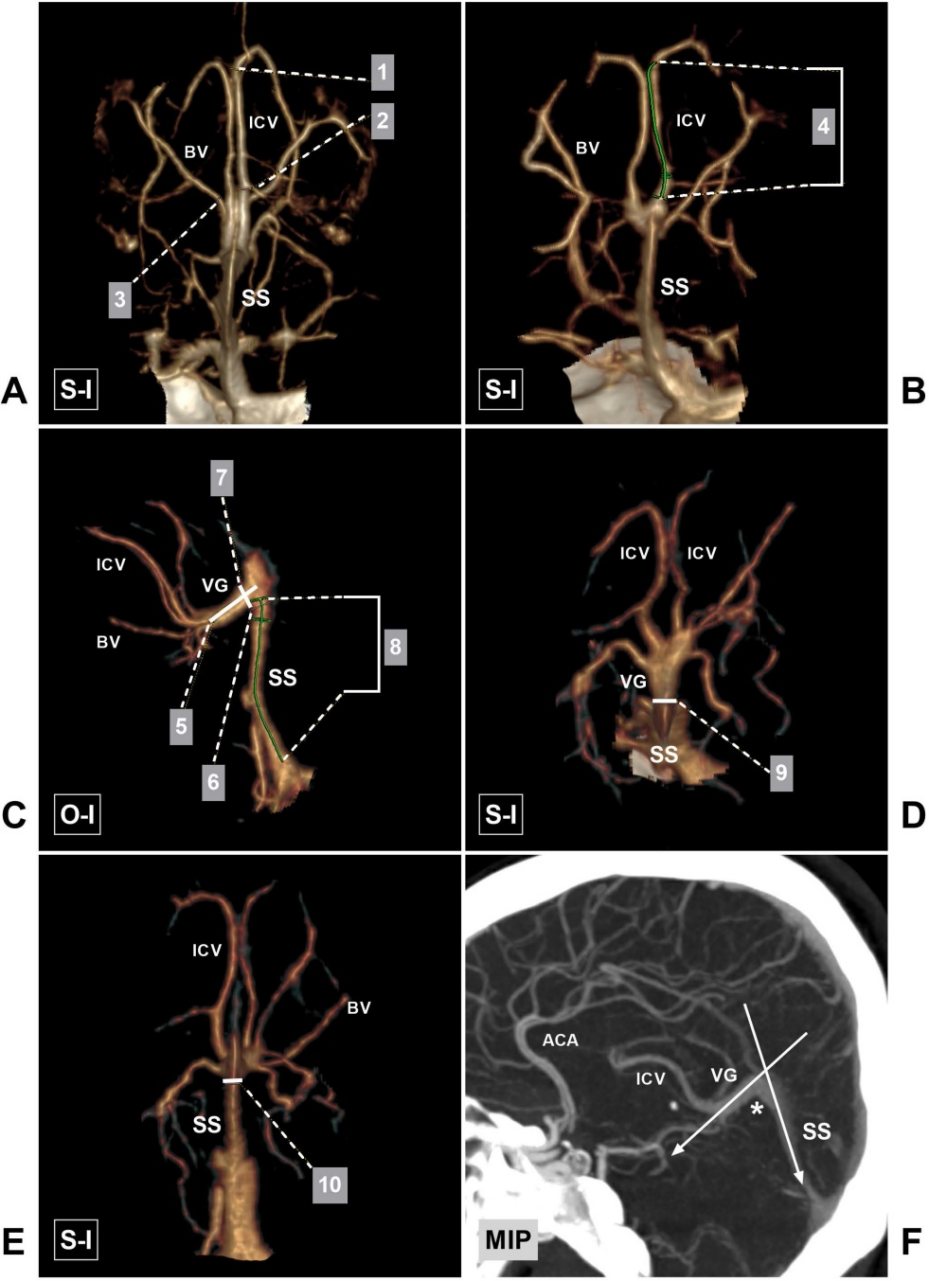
Parameters measured in this study. A, CTA shows the sites at which the diameter of the beginning (1) and termination (2) points of the ICV and the termination (3) point of the BV were measured. B, CTA shows the length (4) of the ICV. C, CTA shows the length (5) of the VG, the width (6) of the beginning of the SS, the thickness (7) of the VG termination point, and the length (8) of the SS. D, CTA shows the width of the VG (9). E, CTA shows the thickness of the beginning of the SS (10). F, CTA shows the angle at the VG and SS transition area (asterisk). Abbreviations: ACA, anterior cerebral artery; BV, basal vein; CTA, computed tomography angiography; ICV, internal cerebral vein; MIP, maximum intensity projection; O-I, outside to inside; S-I, superior to inferior; SS, straight sinus; VG, vein of Galen.
